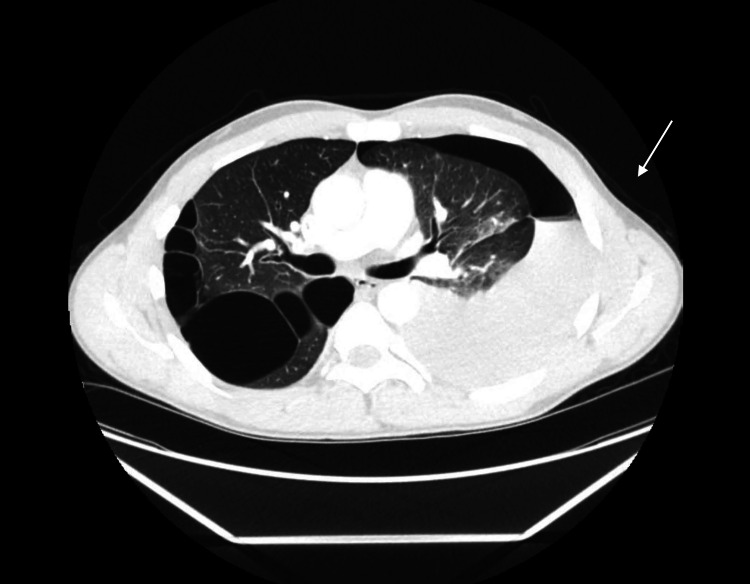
CT showing large left hemopneumothorax with active extravasation of contrast
